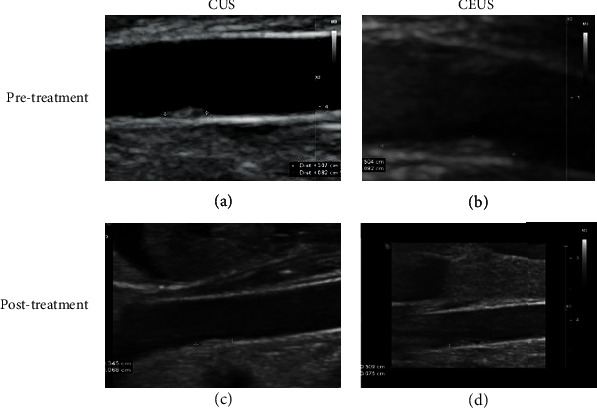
Ultrasound images of a representative plaque in the T2 group in different imaging modes and at different periods. (a) Pretreatment on CUS. (b) Pretreatment on CEUS. (c) Posttreatment on CUS. (d) Posttreatment on CEUS.
